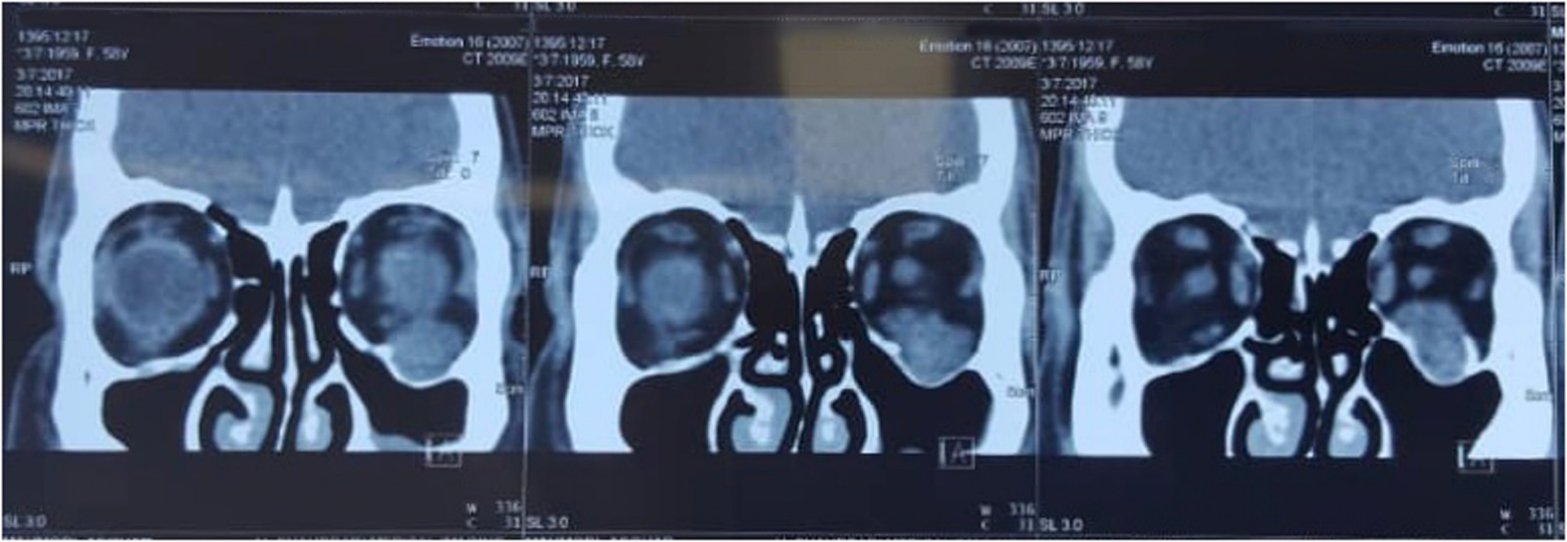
CT showed infraorbital canal enlargement due to homogenous, non‐calcified mass on the left side of coronal section. The posterior wall bowed into the maxillary sinus and the anterior wall rotated to the orbit globe.
